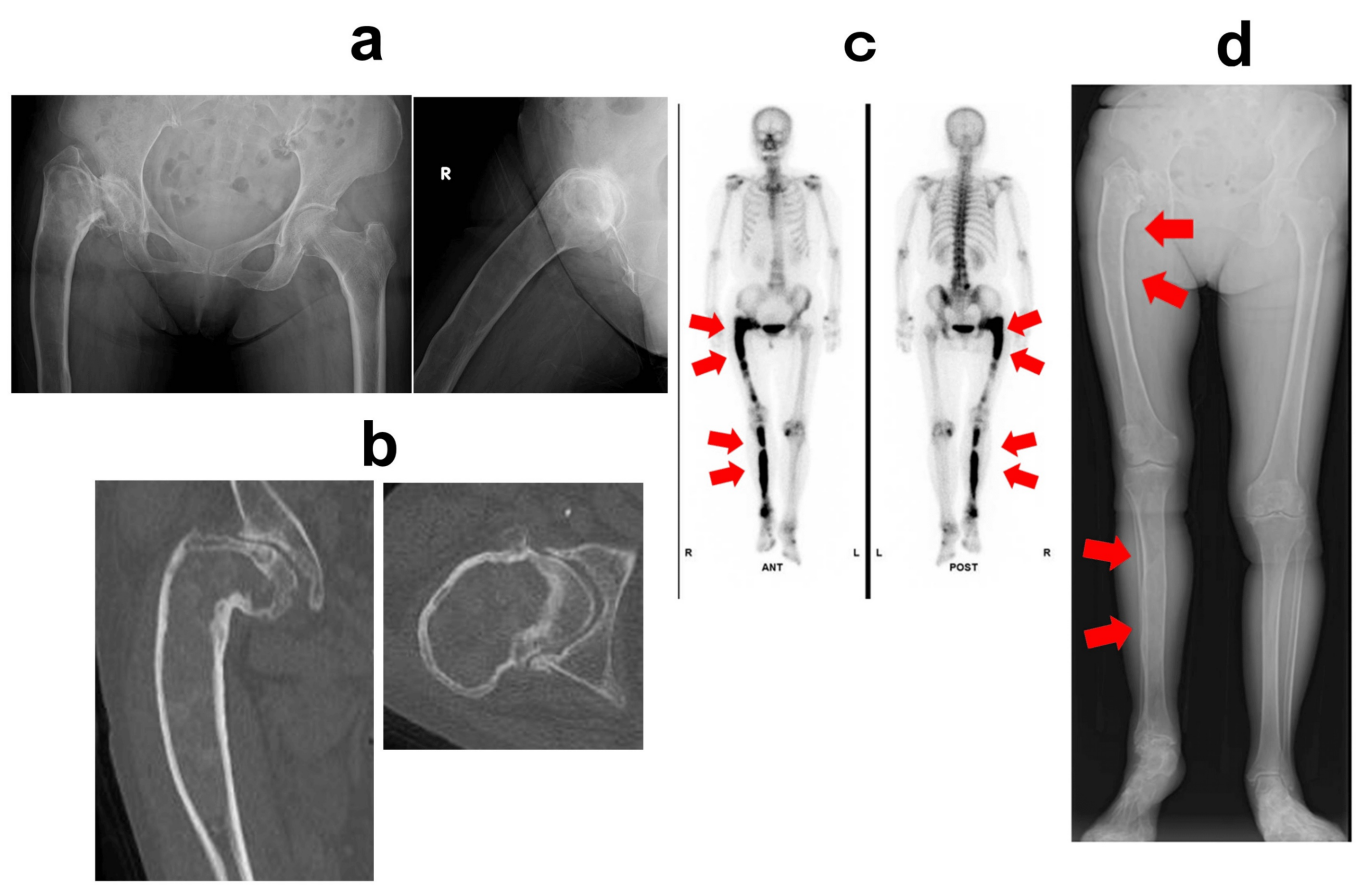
Preoperative imaging assessment (a) Plain radiograph of the hip showing severe bowing deformity of the right femur, with a ground-glass appearance of the medullary cavity and shepherd’s crook deformity with severe secondary osteoarthritis. (b) CT image showing the fibrous lesion within the right femoral medullary cavity. (c) Whole-body bone scintigraphy with technetium-99m indicates increased uptake in the right femur and tibia, consistent with polyostotic FD. (d) Full-length leg radiograph of both lower limbs showing leg length discrepancy. These findings indicate polyostotic FD and secondary osteoarthritis of the right hip. FD, fibrous dysplasia; CT, computed tomography
